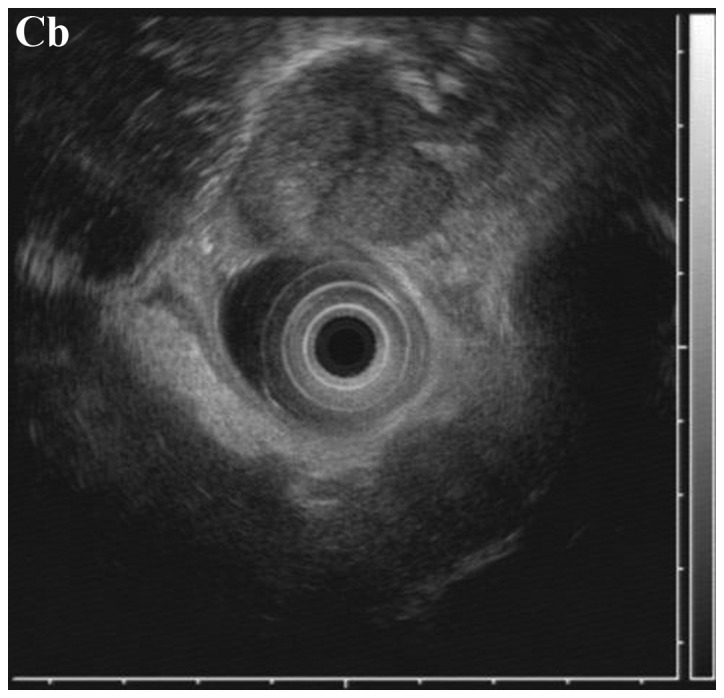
Endoscopic and EUS images of esophageal granular cell tumors. (Aa) A white-to-gray tumor with a smooth surface and (Ab) the EUS image of the same patient showing hypoechoic, homogeneous, smooth-edged lesions derived from mucosal layers. (Ba) A white tumor with a smooth surface and (Bb) the EUS image of the same patient showing hypoechoic, heterogeneous, smooth-edged lesions derived from mucosal layers. (Ca) A pink tumor with a smooth surface and (Cb) the EUS image of the same patient showing hypoechoic, irregular margin and intra- and extra-cavity growth. EUS, endoscopic ultrasound.
